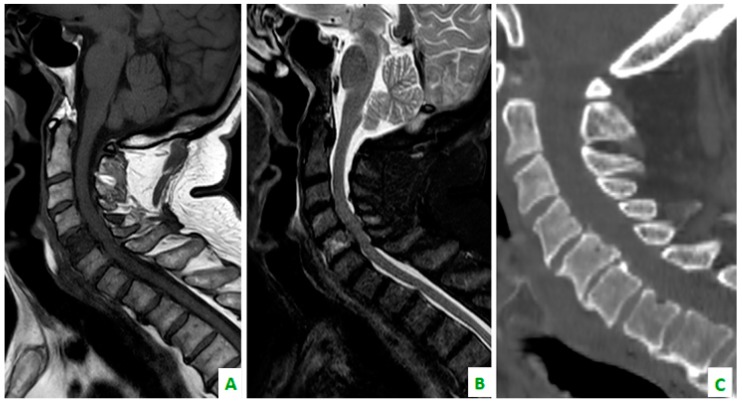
(A) A T1 sequence and (B) STIR sequence, performed with a dedicated spinal MRI, highlight the lung metastases. (C) Cervical spine on a sagittal reconstruction of a computed tomography (CT). The bone metastasis is not so clear.
